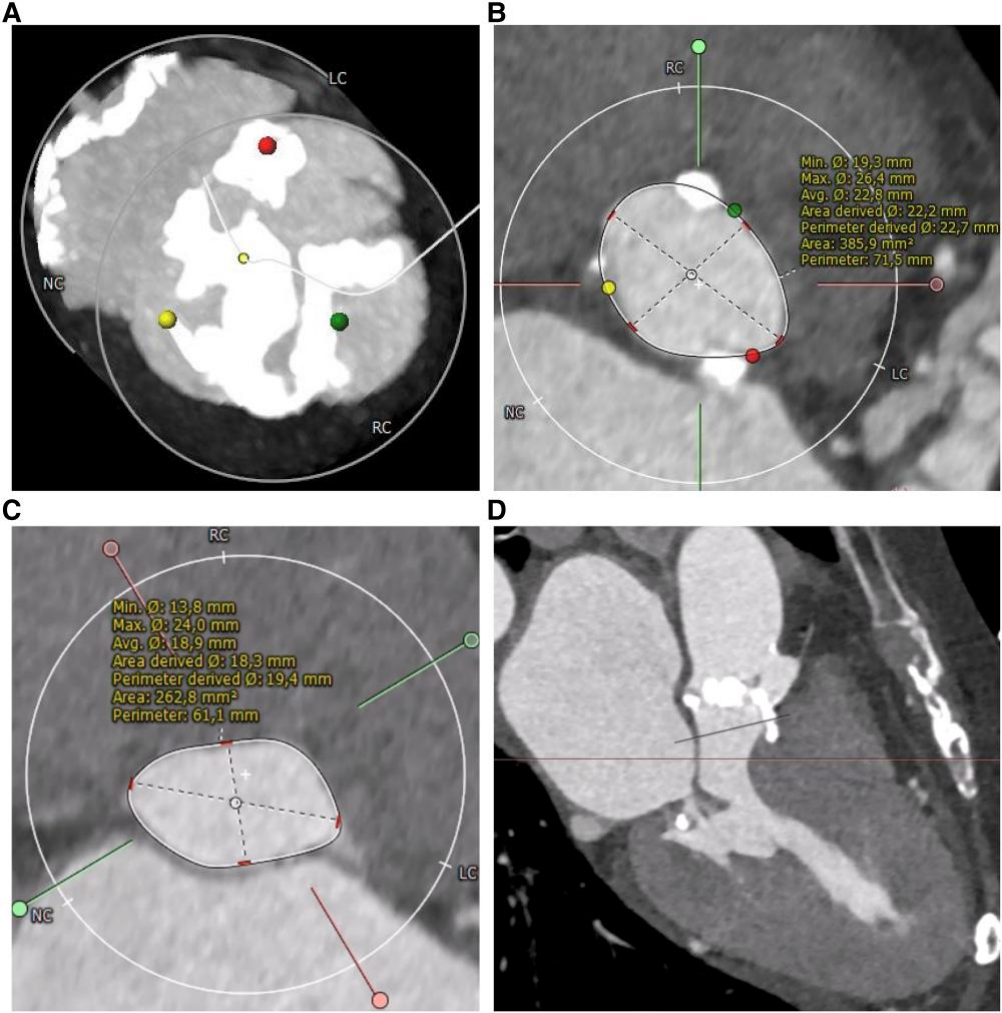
Pre-operative end-systolic computed tomography of the heart. (*A*) Computed tomography scan of the aortic valve shows severe calcification. (*B*) Annular assessment. (*C*) Assessment of the left ventricular outflow tract (LVOT) 8.5 mm below annular plane. (*D*) Left heart section showing left ventricular hypertrophy and small left ventricular chamber.
